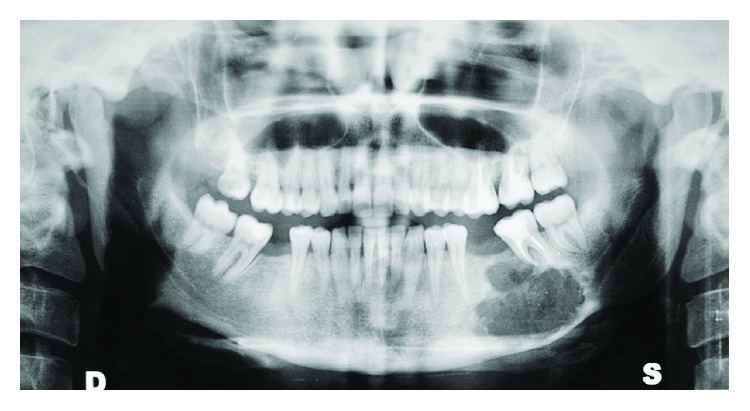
Panoramic X-ray before the excision of ossifying fibroma.
